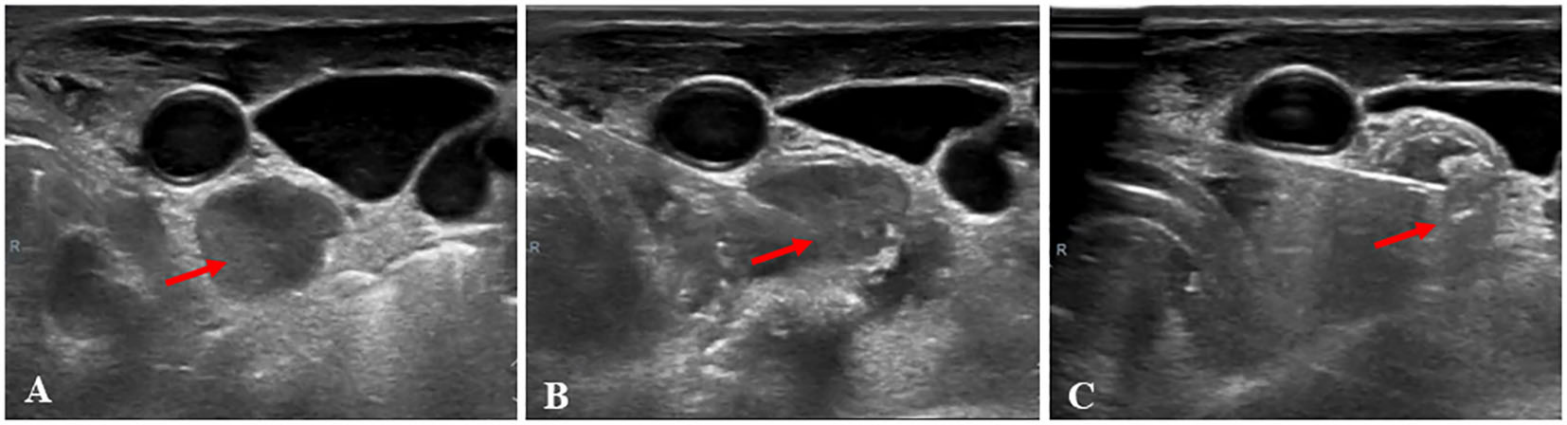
A 48-year-old woman, who had a total thyroidectomy three years ago due to papillary thyroid cancer, received microwave ablation (MWA) for cervical metastatic lymph nodes. (A), Prior to MWA treatment, B-mode ultrasonography (US) showed a hypoechoic LN (arrow); (B), The LN was punctured with a MWA needle (arrow); (C) During MWA, a hyperechoic pattern (arrow) was observed in the LN.
